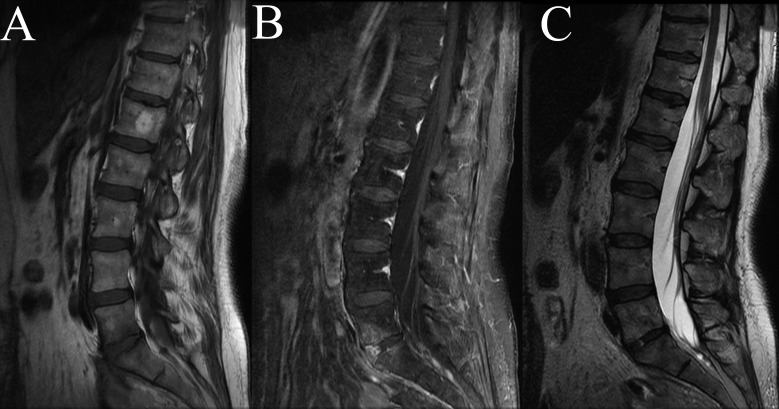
MRI of Lumbar Spine after Antibiotics Figure 3: Sagittal MRI of lumbar spine six weeks after completing a twelve-week antibiotic course with T1 (A), contrasted T1 (B) and T2 (C) sequences showing absence of previous T2 hyperintensity and enhancing scar anterior to L5-S1.
